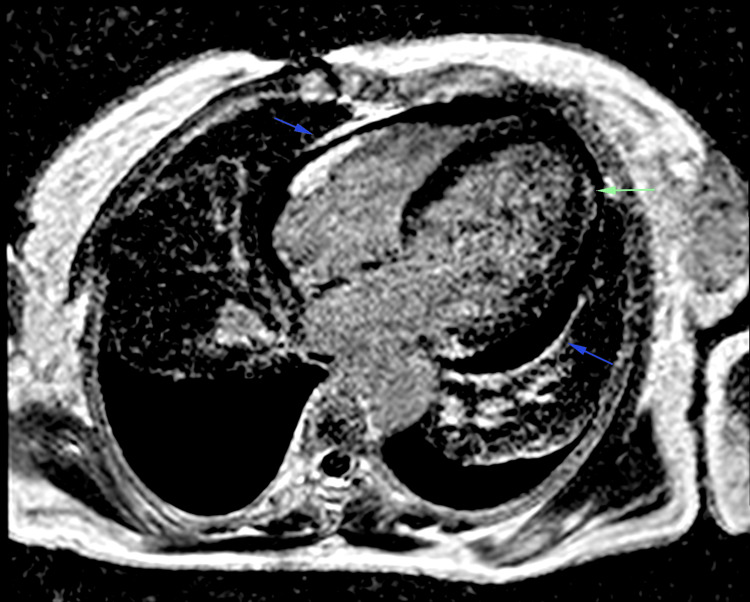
STIR MRI sequence in a four-chamber view showing late enhancement in the subepicardial medio-lateral walls (indicated by green arrow) and pericardial leaflet (indicated by blue arrows). STIR MRI: short-tau inversion recovery magnetic resonance imaging
